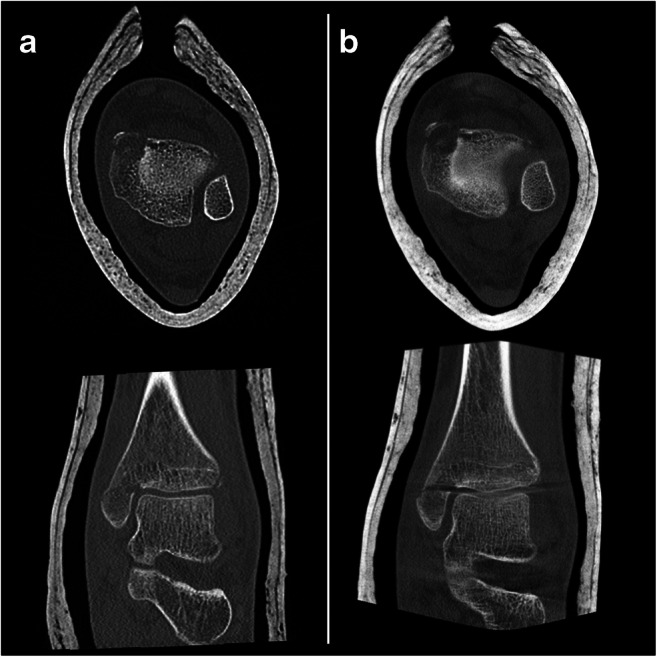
A side-by-side comparison of an MDCT examination of the right ankle in a 15-year-old girl with a distal tibial fracture. a Axial (top) and coronal (bottom) MDCT images. b Axial (top) and coronal (bottom) reconstructions of the corresponding CBCT examination. Especially the coronal slices show beam hardening artifacts in the image plane at bone-to-cartilage transitions
